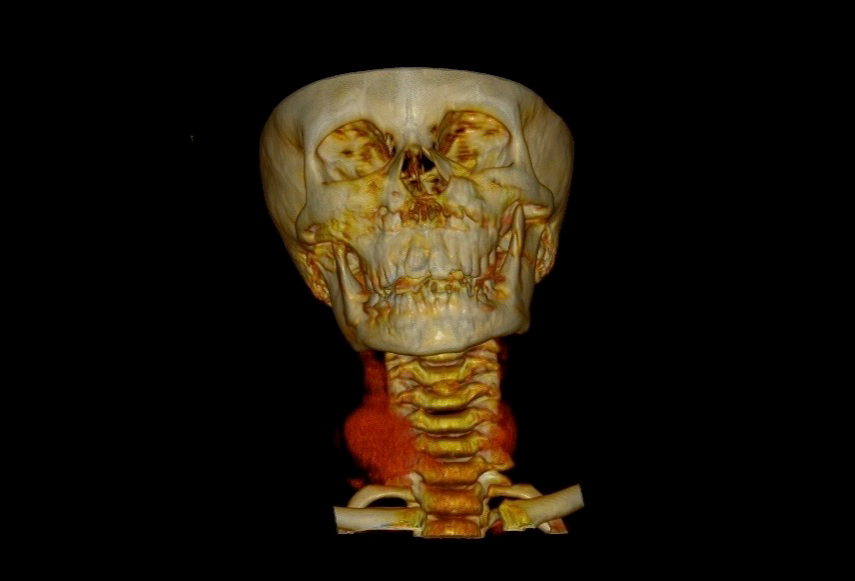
Cervical CT in coronal plane with 3D vascular reconstruction.
